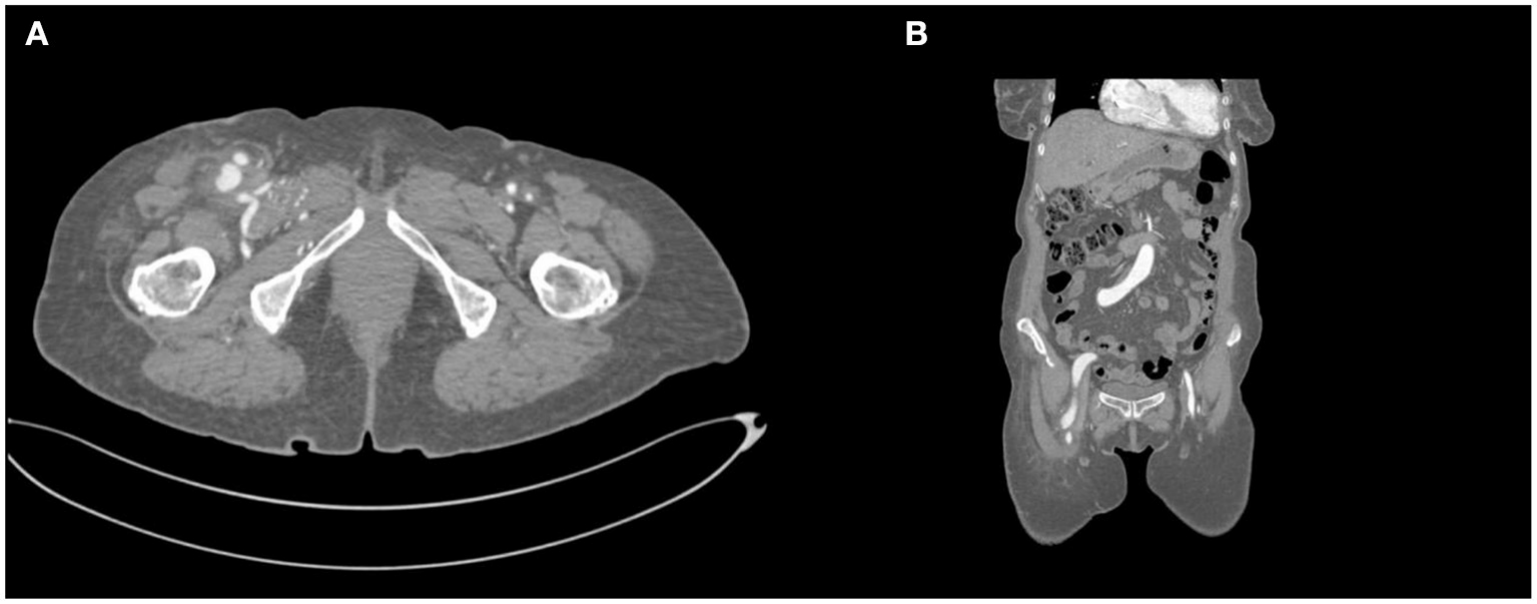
CT angiography after the operation presenting: (A) axial section, femoral artery bifurcation lack of contrast in the femoral vein and (B) coronal section, femoral artery bifurcation- lack of contrast in the femoral vein.
